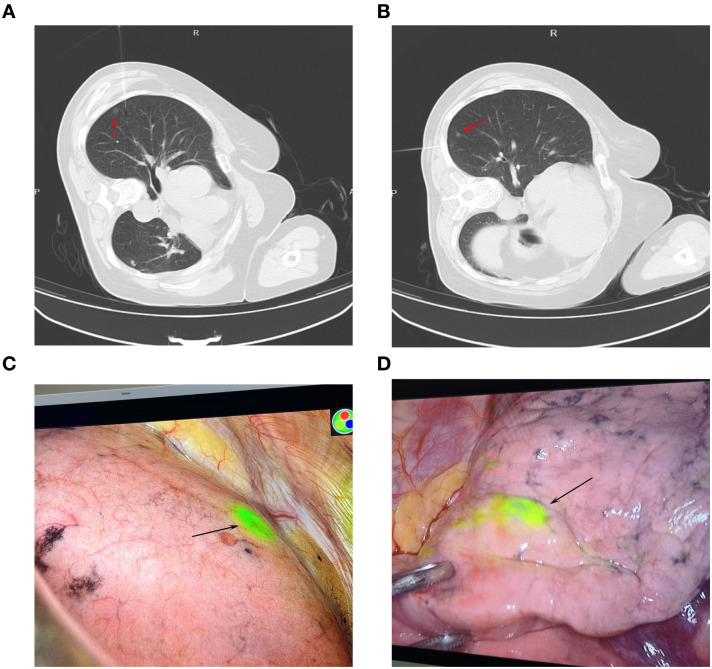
The CT-guided IG localization for PNs (arrows) in the right (A) upper and (B) lower lobes. (C, D) The IG localization (arrows) was found in the lung surface during the VATS.
